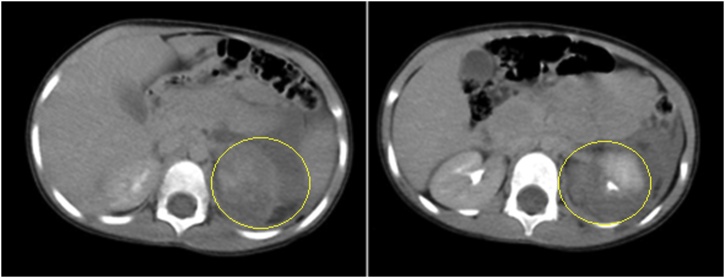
Case 1, CT trauma of the revealed shattered left kidney with huge perinephric and retroperitoneal hematoma (yellow circle).
